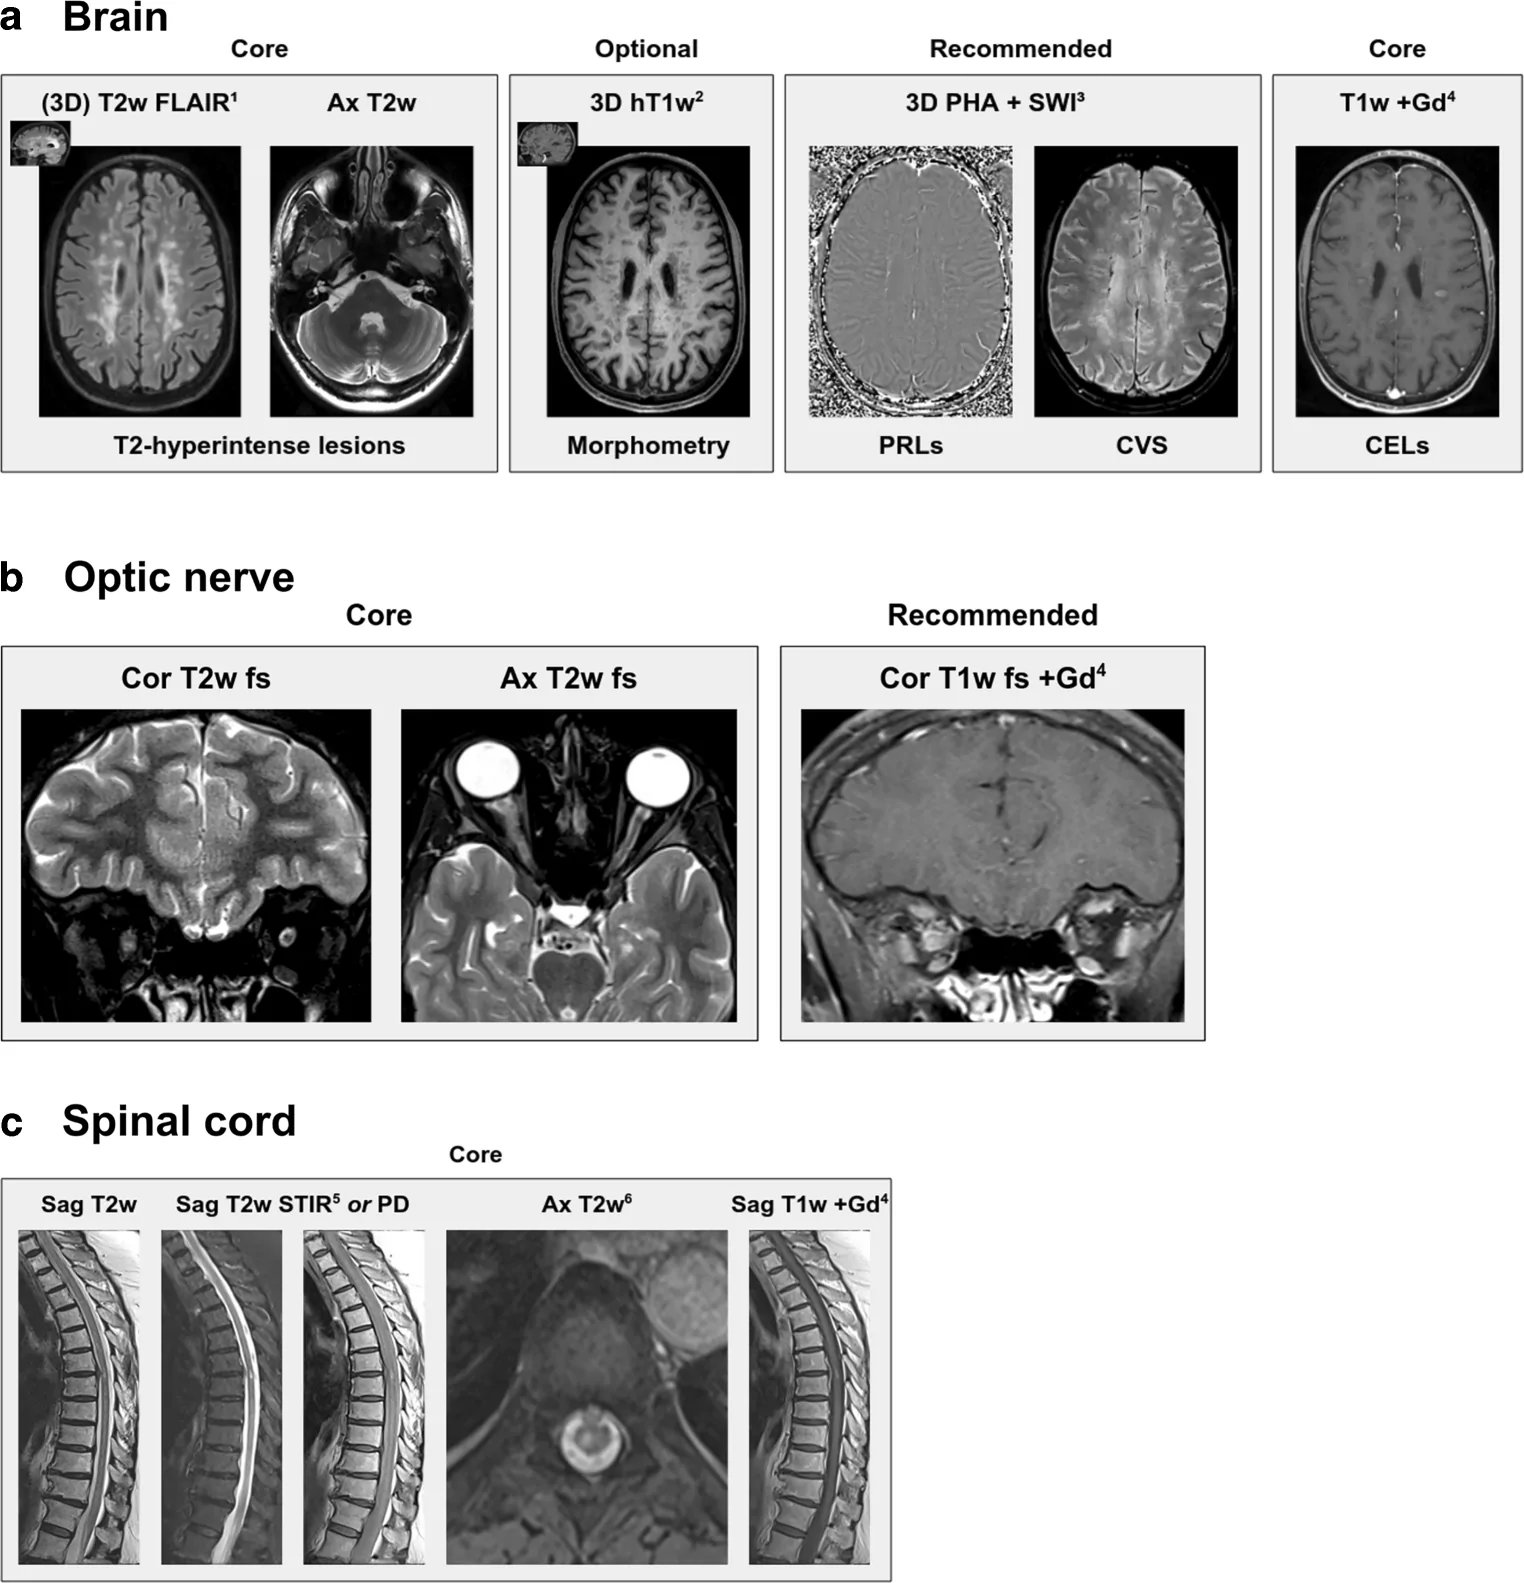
Overview of protocol recommendations for brain (a), optic nerve (b) and spinal cord (c). ^1^ 3D T2w FLAIR imaging is strongly recommended. Fat suppression is optional, but recommended. ^2^ Heavily 3D T1w is recommended for centers that want to include atrophy measurements for research purposes. ^3^ In cases outlined in the section “Diagnostic algorithm”, SWI is strongly recommended to be acquired. ^4^ Standard dose of 0.1 mmol/kg body weight for established macrocyclic GBCA. For next-generation GBCAs, the efficacy of potentially lower doses remains to be prospectively validated. Minimum delay before T1w image acquisition of 5–7 min. ^5^ T2w STIR should not be acquired after GBCA application. ^6^ Cover the entire spinal cord.
